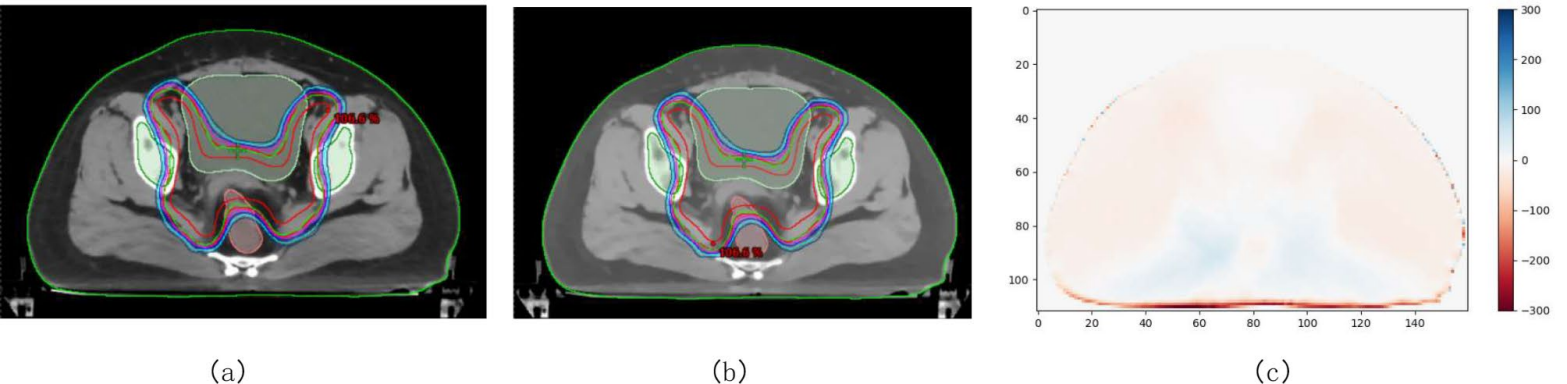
Photon dose distribution in an example pelvic patient (Patient 2). (a) Treatment plan based on the conventional CT image. (b) Treatment plan based on the ED map. (c) Difference map of dose distribution between the two treatment plans. ED, electron density
